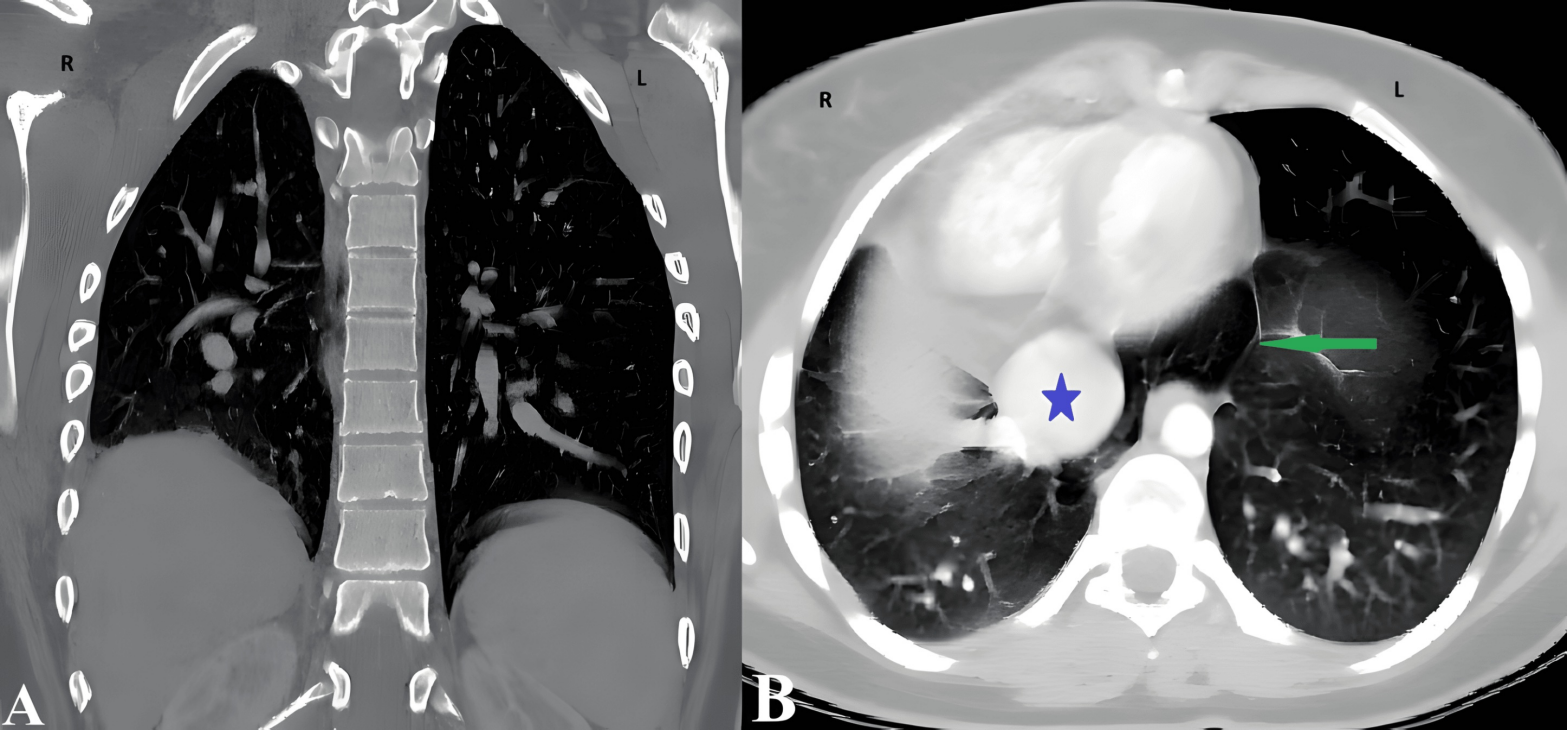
Coronal (A) and axial (B) CT of the chest (lung windows) An overall hypovolemia of the right lung (hypoplastic lung), with compensatory hypervolemia of the left lung. Well-aerated, retro-cardiac, tongue-like projection (green arrow) of the right lung crossing the midline (horseshoe lung), with a dilated, congested inferior vena cava (blue star). Labels: R = right lung, L = left lung.
